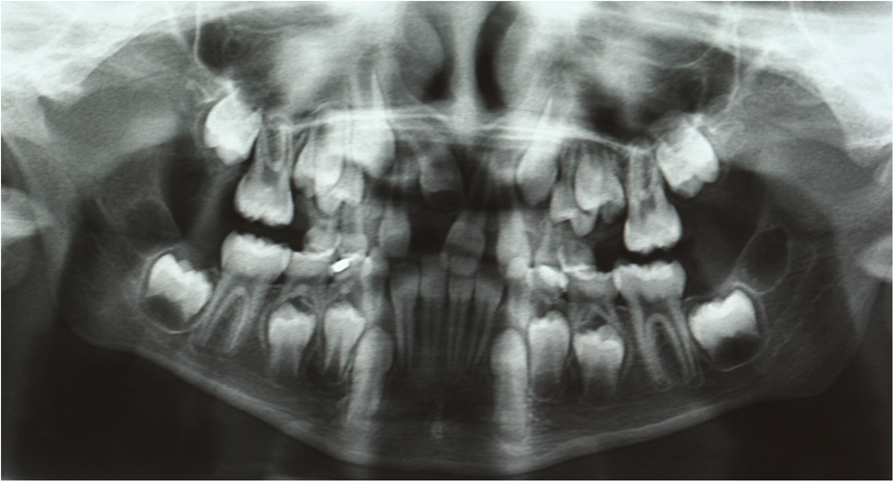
Initial panoramic radiography.
